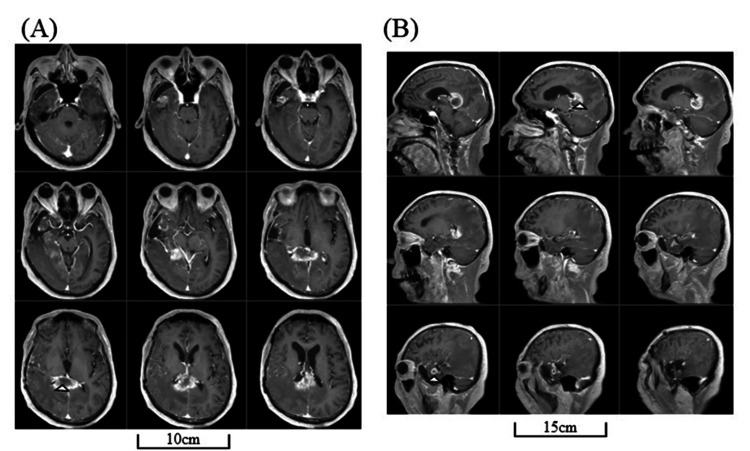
MRIs at recurrence Head MRIs at the recurrence revealing new enhanced lesions at the right residual anterior STG and MTG, hippocampus, and splenium of corpus callosum by axial (A) and sagittal (B) view of post-enhanced T1-weighted images (arrowheads). MRI: magnetic resonance imaging; MTG: middle temporal gyrus; STG: superior temporal gyrus
